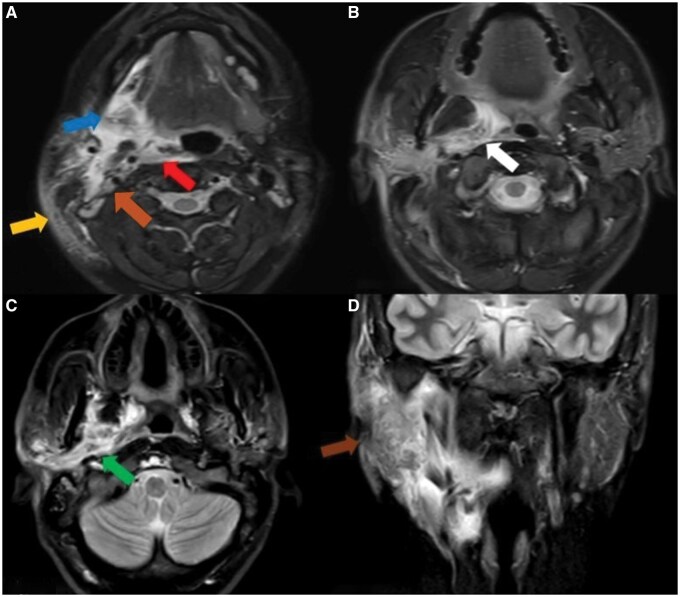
Fat-suppressed T2-weighted MR images demonstrating the multidirectional spread of the deep neck infection. (A) Axial fat-suppressed T2-weighted image showing subcutaneous extension (yellow arrow), involvement of the submandibular space (blue arrow), retro-pharyngeal spread (red arrow), and carotid space extension (orange arrow. (B) Axial fat-suppressed T2-weighted image depicting para-pharyngeal compartment involvement (white arrow). (C) Axial fat-suppressed T2-weighted image demonstrating spread into the masticator space (green arrow). (D) Coronal fat-suppressed T2-weighted image showing extension into the parotid compartment (brown arrow).
